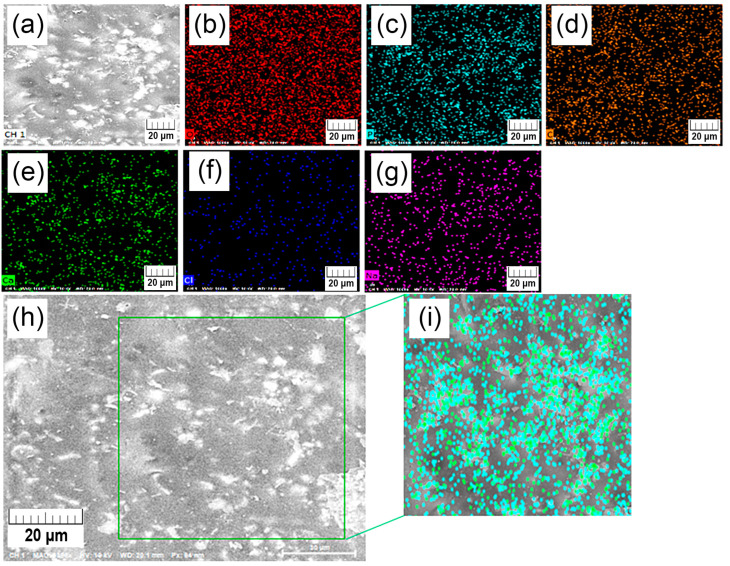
An energy-dispersive X-ray (EDX) analysis of the PLA-20-HAp surface after 10 weeks of in vitro degradation (a). Figures represent the distribution of oxygen (b), phosphorous (c), carbon (d), calcium (e), chlorine (f), and sodium atoms (g) on the same surface area. In the bottom detailed topography of surface (h) the topography of phosphorus (turquoise) and calcium (green) atoms is shown separately (i). Scale bars represent 20 µm.
